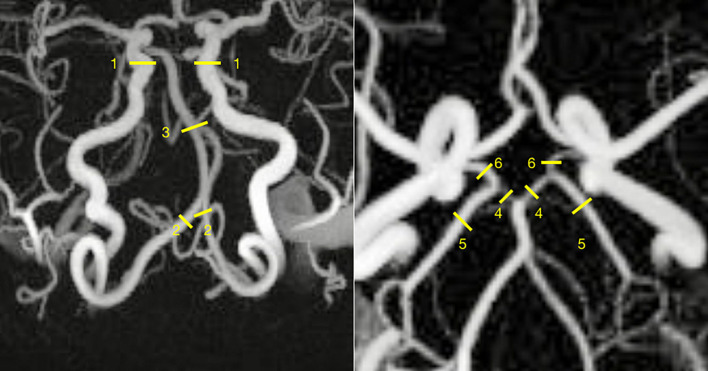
Sample 4D flow MRI complex difference angiogram. Flow was measured in the internal carotid arteries (1), vertebral arteries (2), basilar artery (3), P1 and P2 segments of the posterior cerebral artery (4, 5), and the posterior communicating arteries (6).
